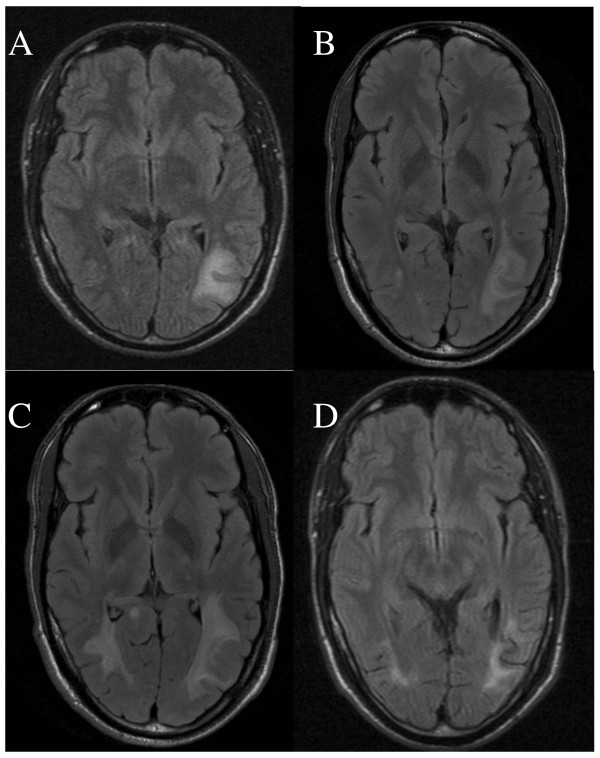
MRI on admission and follow-up. A. MRI (flair T2) performed on admission showing a white matter lesion in the parieto-occipital region on the left side. B. MRI (flair T2) showing progression of the white matter lesion 10 days after admission. C. MRI (flair T2) showing progression of the white matter lesions in both hemipheres 3 1/2 weeks after onset of treatment with cidofovir. D. MRI (flair T2) shower regression of the white matter lesions in both hemispheres 6 months after onset of treatment with cidofovir.
